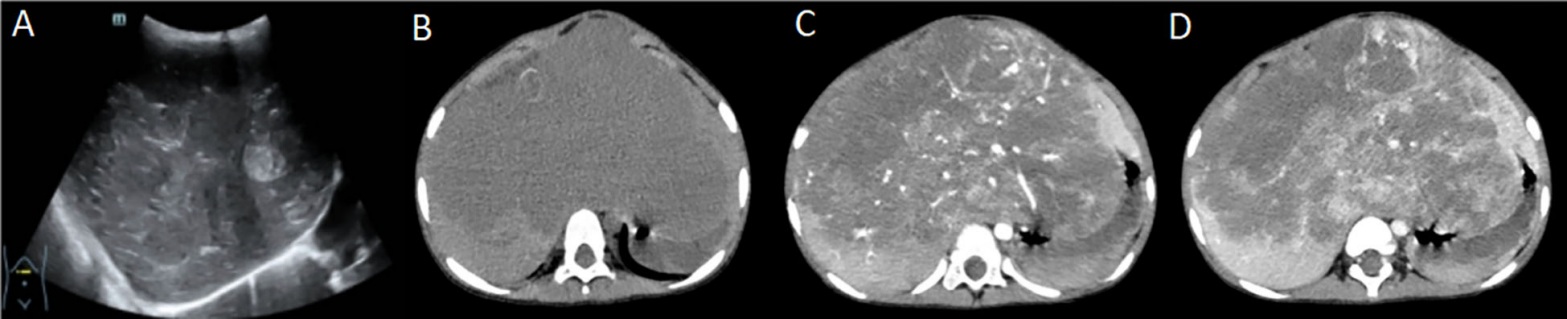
(A) Liver ultrasound; (B) Liver computed tomography (CT) - plain image; (C) Liver CT enhancement scan - arterial phase; (D) Liver CT enhancement scan - portal venous phase (part of the portal venous system is not well displayed due to tumor encapsulation).
